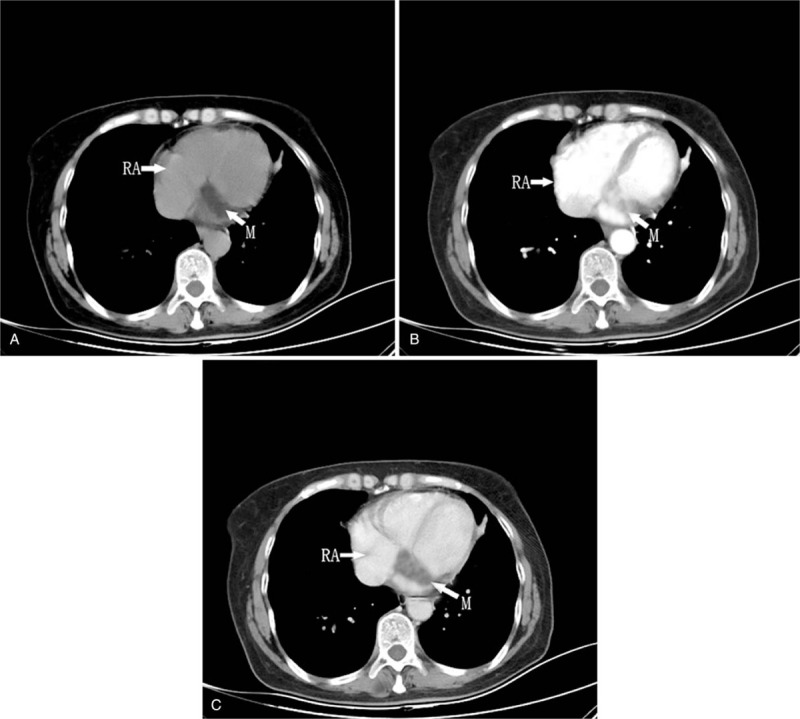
(A) Computed tomography of a low-attenuating lesion in the coronary sinus before contrast agent injection. (B) The tumor displayed heterogeneous moderate enhancement during the arterial phase. (C) Heterogeneous mild enhancement of the tumor in the delayed phase. M = mass, RA = right atrium.
